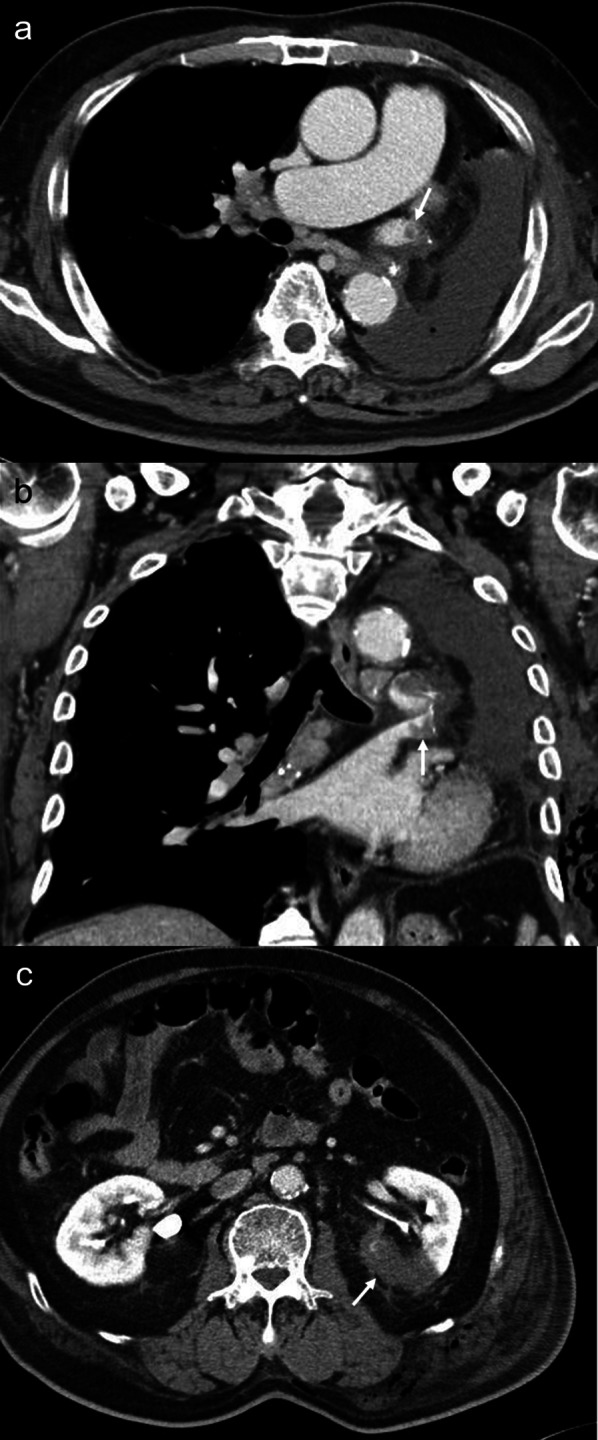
Contrast-enhanced computed tomography reveals a thrombus in the left upper pulmonary vein stump: a coronal view, b horizontal view, and c partial defect of the left kidney on postoperative day 4
